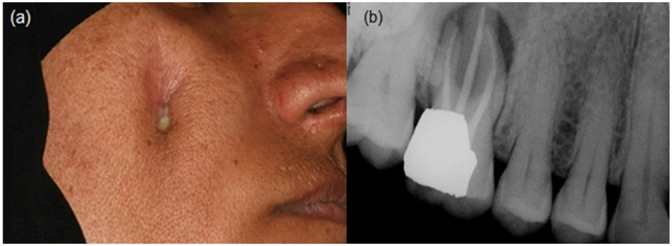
Radiograph and photograph of the patient in the examination session: a: Lateral view showing discharge. b: A periapical radiograph showing tooth #16 with radiolucency and complete root canal filling.
